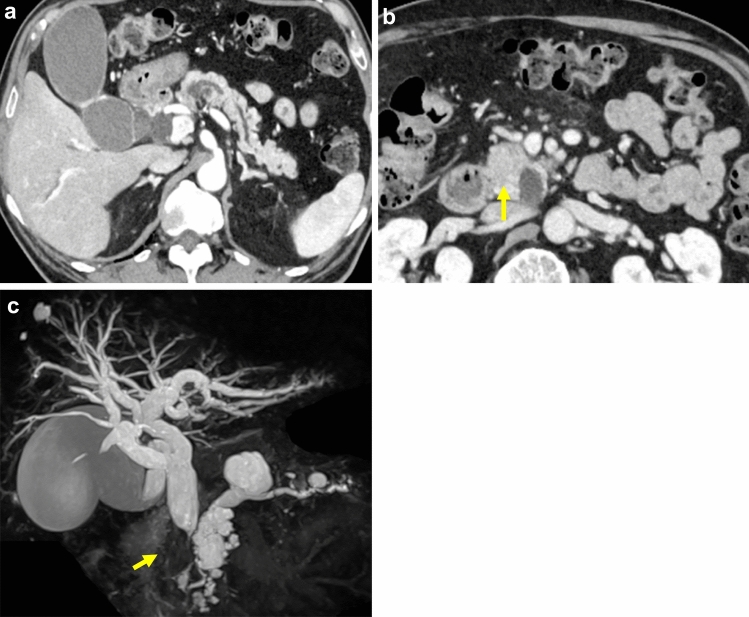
CT and MRCP findings. a Dilation of the main pancreatic duct is seen. b Tumor of mild low density in the groove region of the pancreatic head (arrow). c Stenosis of the bile duct due to a pancreatic tumor (arrow) and branched pancreatic duct-type IPMN. *IPMN* intraductal papillary mucinous neoplasm; *CT* computed tomography; *MRCP* magnetic resonance cholangiopancreatography
